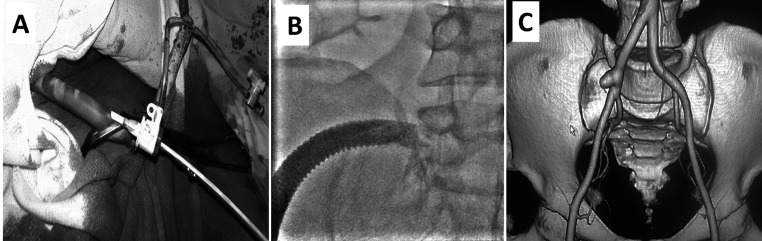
The Polytetrafluoroethylene (PTFE) graft (A) anastomosed with the right common iliac artery (CIA) (B) was visible. A follow-up 3-dimensional volume-rendered computed tomography (CT) angiographic image showed a small conduit stump attached to the right CIA (C).
